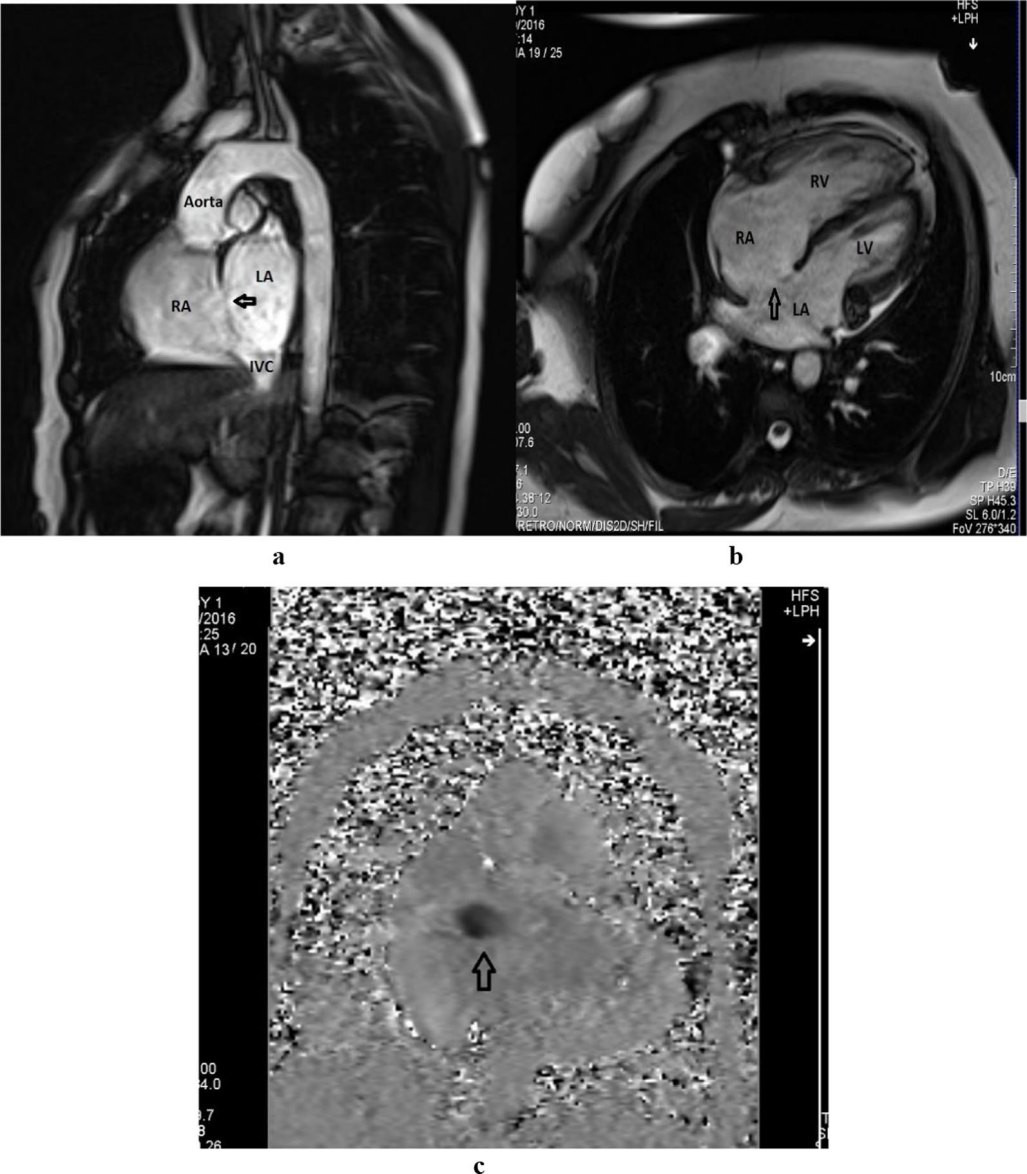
Phase contrast CMR images depicting ASD (arrow heads) in Oblique Sagittal view (a), Axial/Four chamber view (b), and *enface* view (c). Note: ASD, Atrial Septal Defect; CMR, Cardiac Magnetic Resonance Imaging
